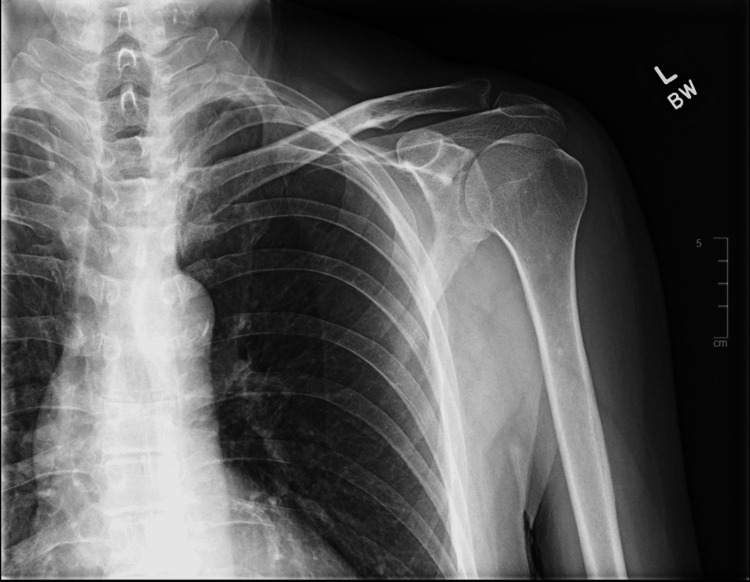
X-ray of the left shoulder No acute fracture or posttraumatic left shoulder dislocation. Acromioclavicular and coracoclavicular intervals are maintained. Mild osteoarthrosis of the left shoulder with spurring about the acromioclavicular joint.
